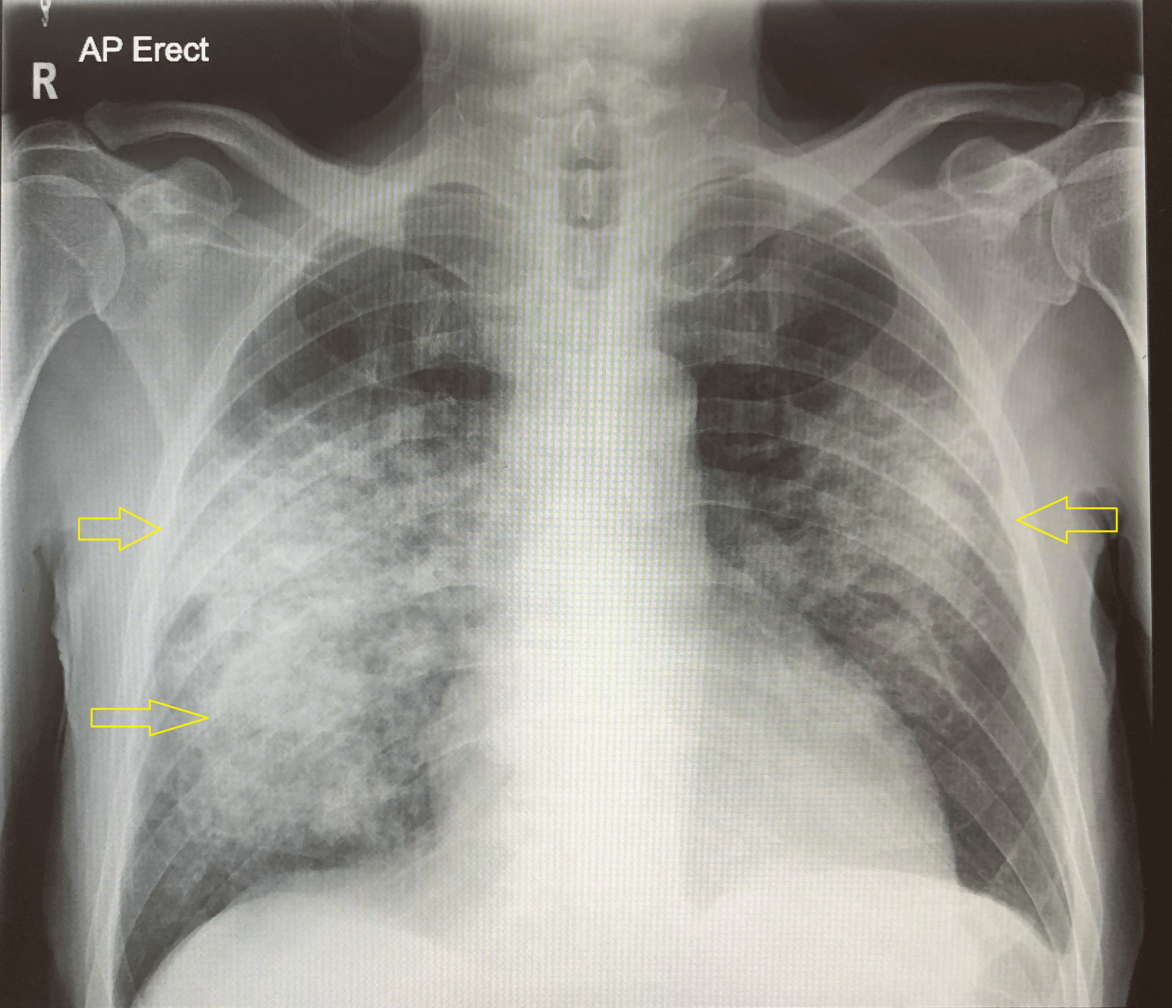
Chest X-ray showing bilateral widespread multifocal airspace shadowing, more prominent on the right. Trivial blunting of the left costophrenic angle.
